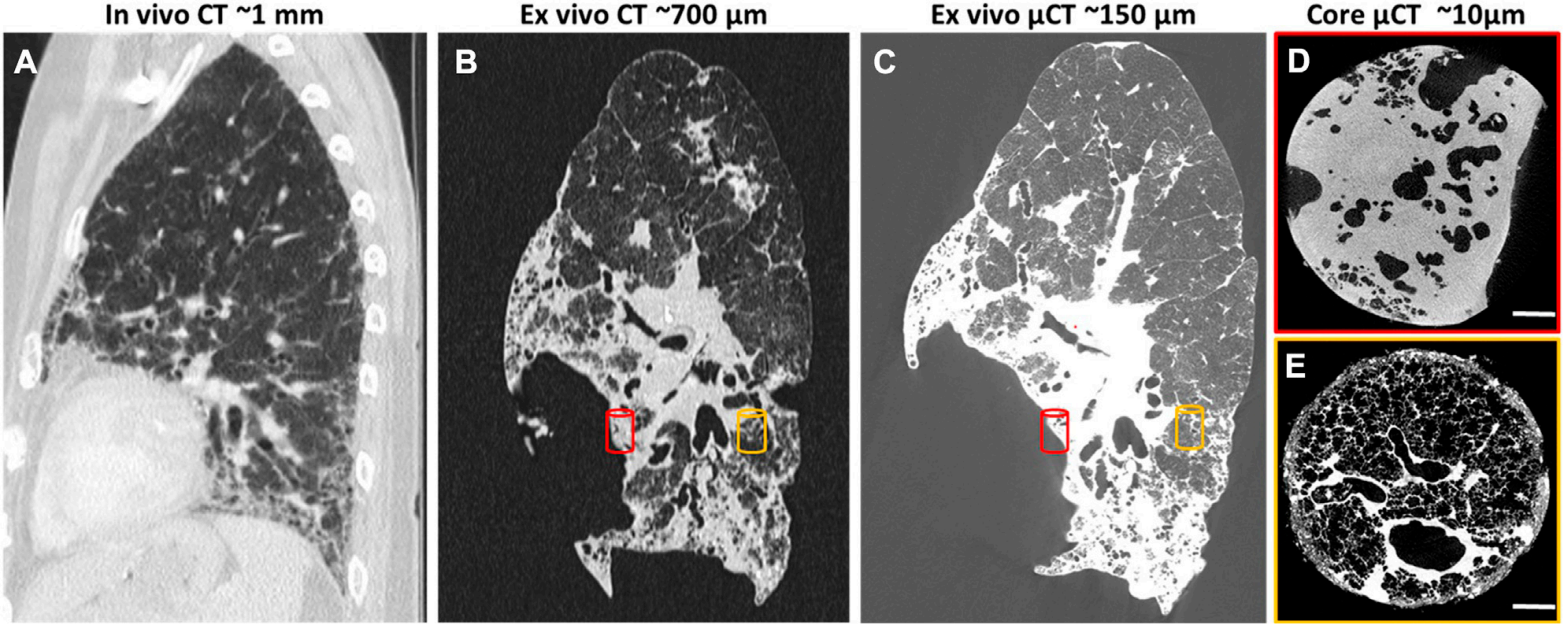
HRCT scans of human lung with usual interstitial pneumonia (UIP)[reprinted with permission from Tielemans et al. (2020)] (A)
